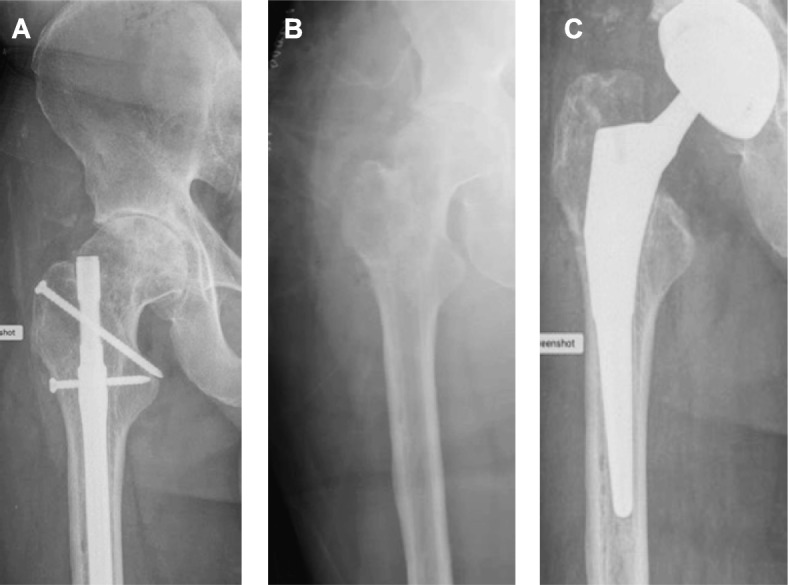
Radiographic images of patient #1 before and after THA. a Pre-operative radiograph showing advanced hip osteoarthritis and a femoral nail from previous fracture approximately 20 years prior. b Post-operative radiograph from the first procedure (Stage 1) after the femoral nail was removed and the void was filled with V-CBA to rebuild the bone stock. c Post-operative radiograph from second procedure (Stage 2) showing the hip replacement hardware. The second procedure was performed 7 weeks after the first procedure
